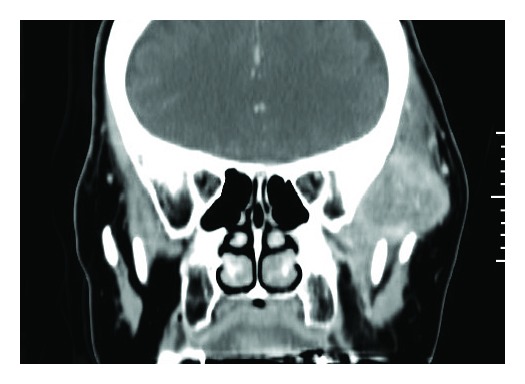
Preoperative contrast CT showing ill-demarcated low-density mass with enhancement of the adjacent temporal muscle.
